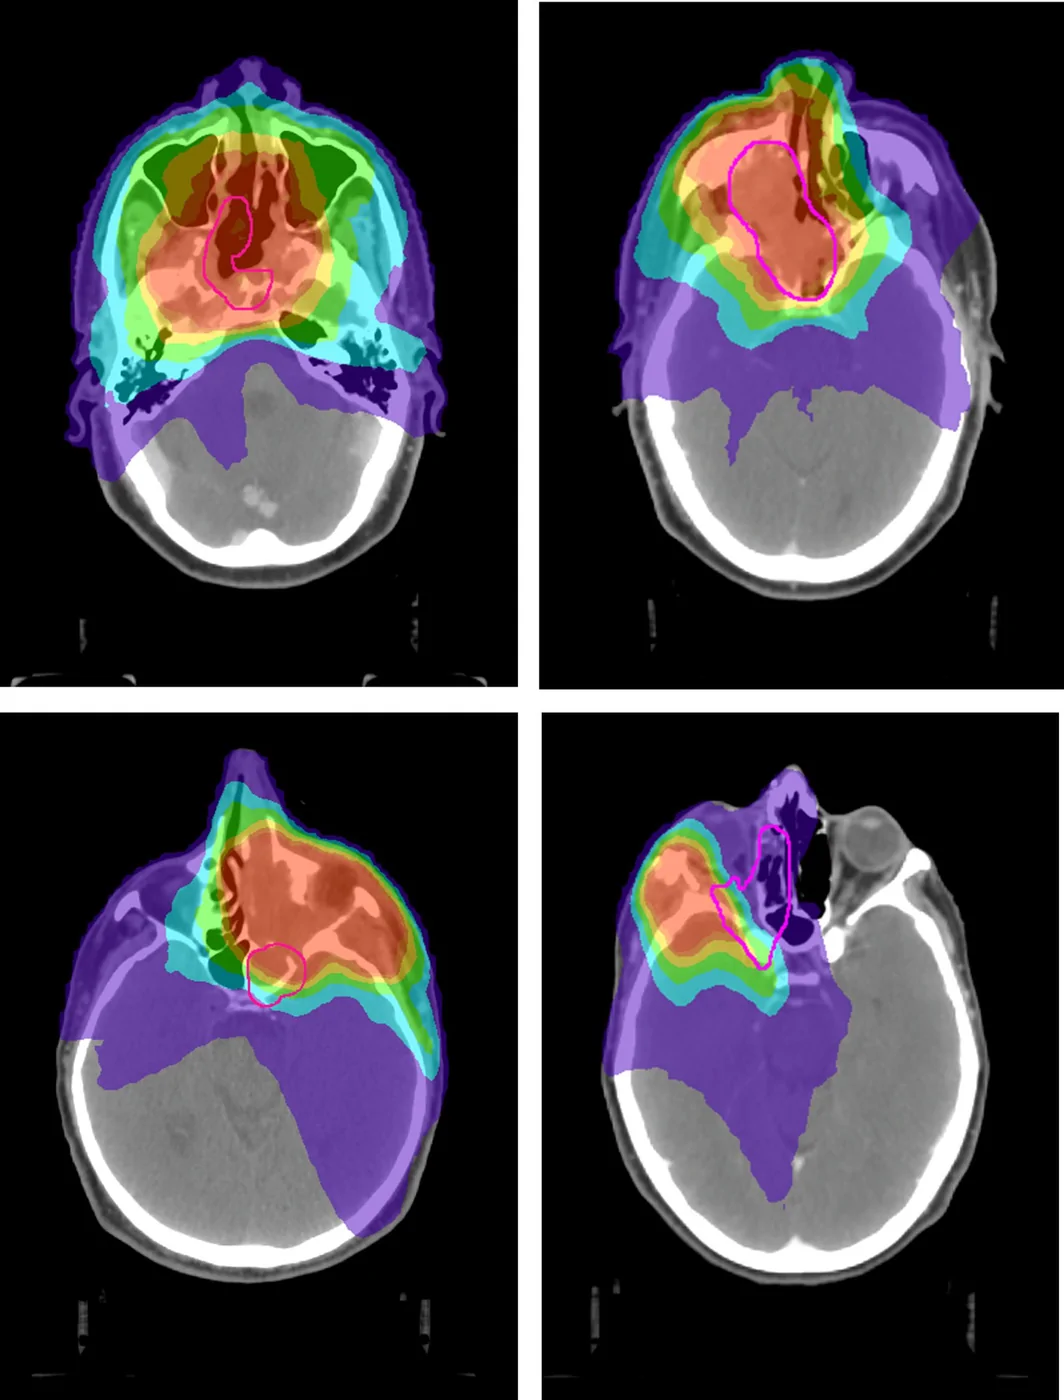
Four examples of recurrences (pink) with on the initial CT‐scan with dose overlay (red = 95% isodose). Top row 2 patients with in‐field recurrences. Bottom row 2 patients with marginal (left‐hand side) or out‐of‐field (right‐hand side) recurrences. [Color figure can be viewed at wileyonlinelibrary.com]
